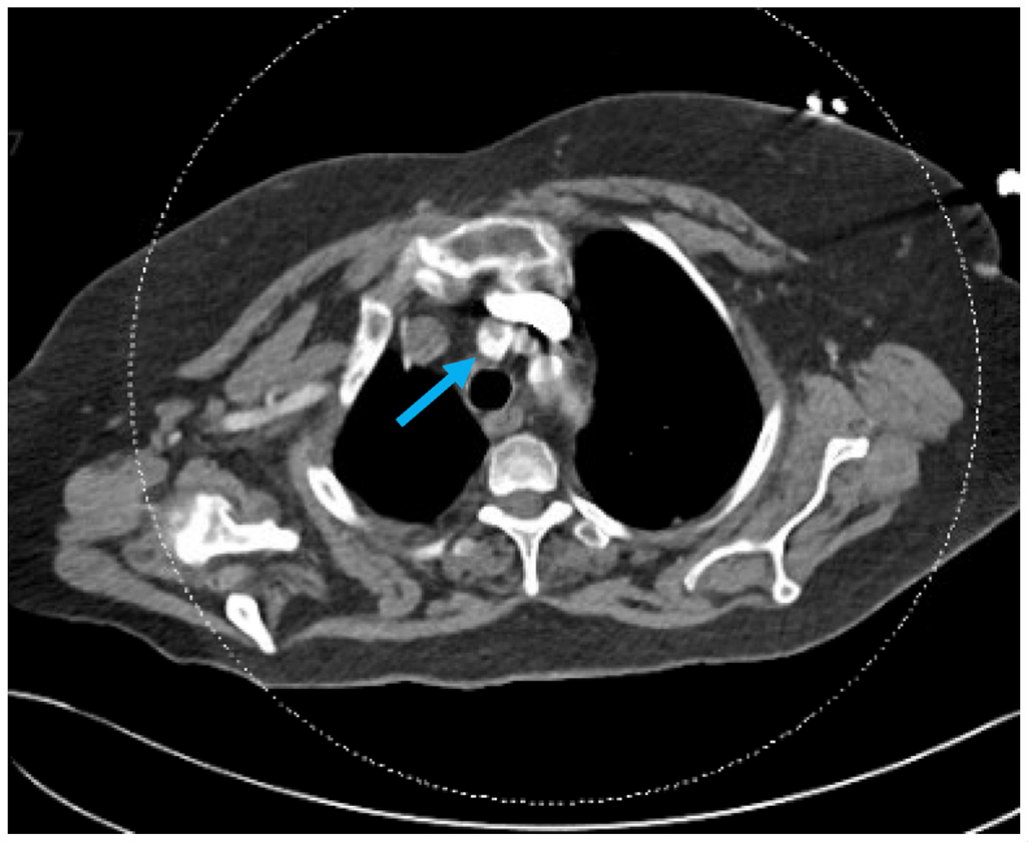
Computed tomography angiography (*CTA*) with contrast showing nonocclusive thrombus in the innominate artery from its origin to just proximal to its bifurcation (*blue arrow*).
